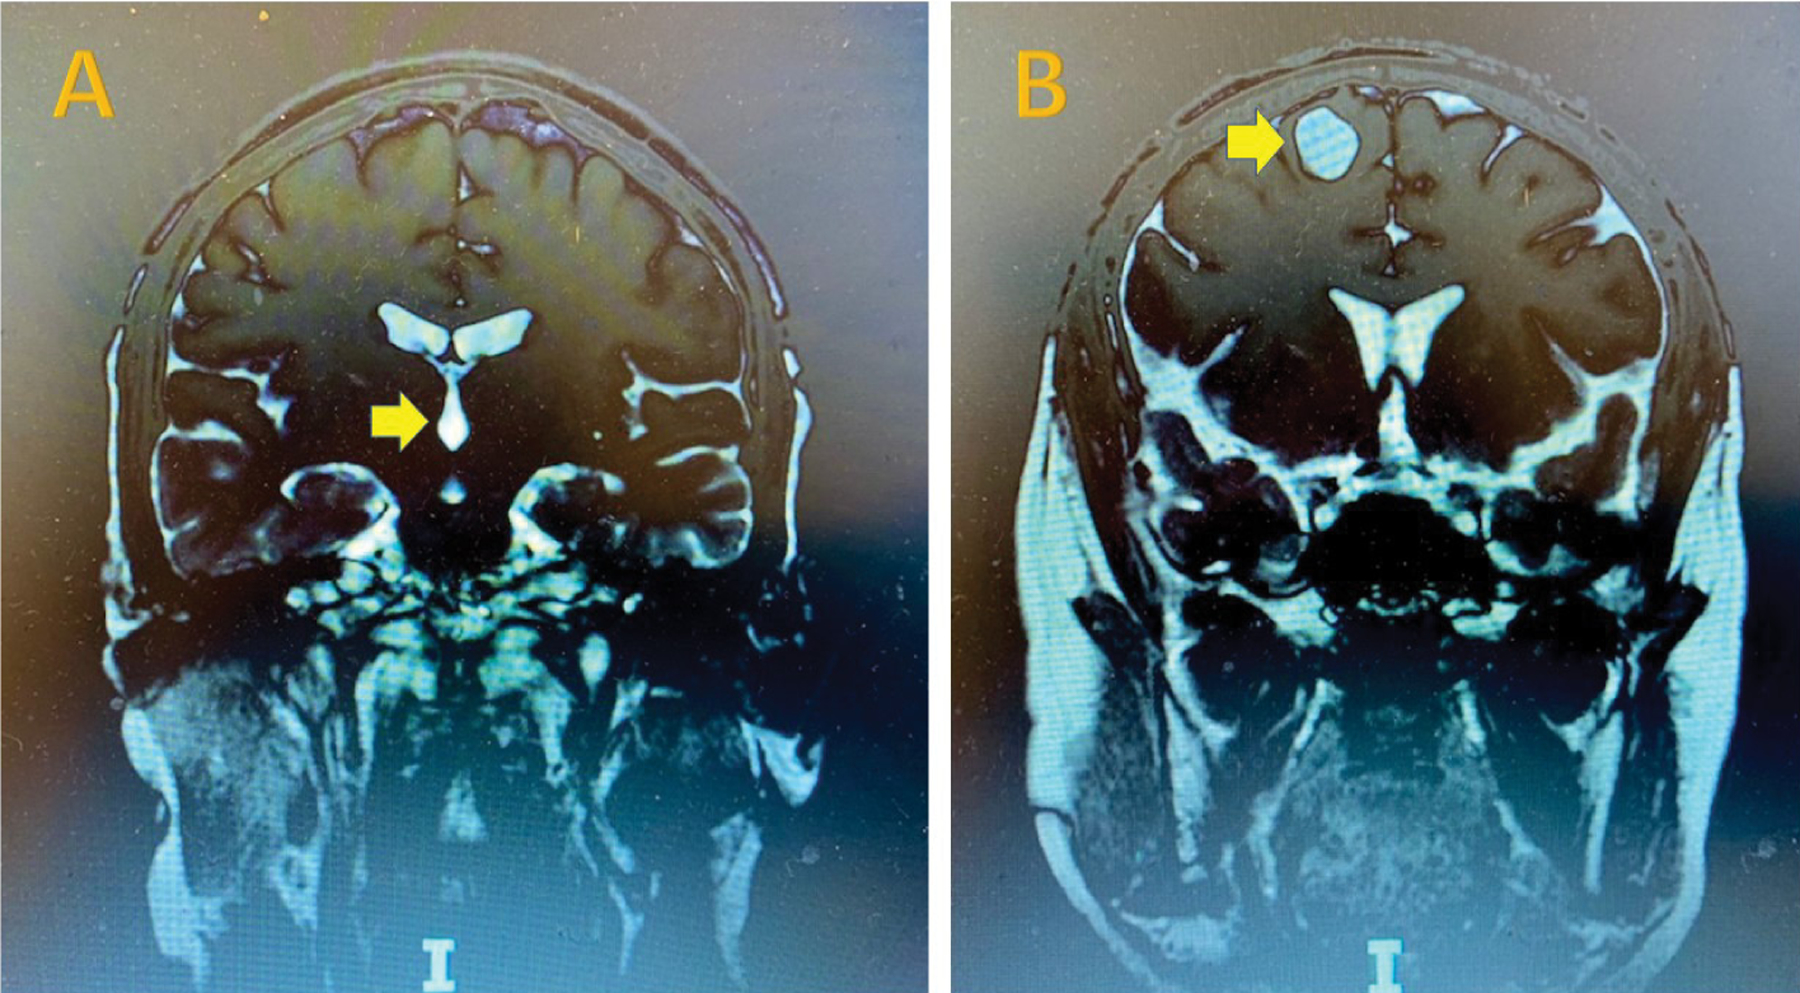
Cystic lesions on MRI. 4^th^ ventricle and right frontal lobe scoleces in cysts with arrows.
